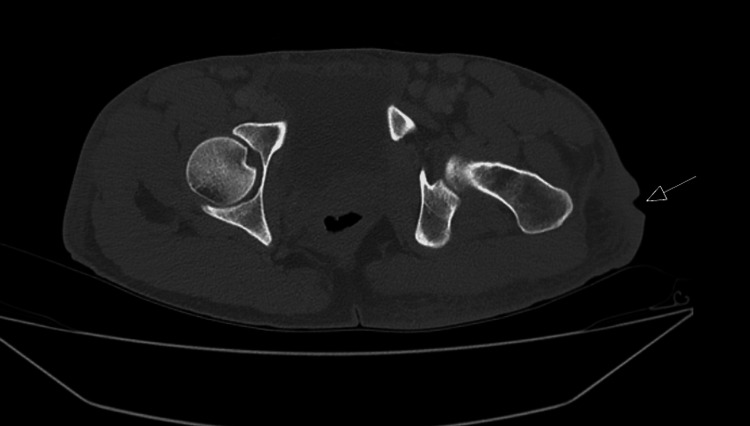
CT scan of the abdomen and pelvis showing superficial infection of the left lateral hip without osteomyelitis. The arrow indicates the area of infection.
